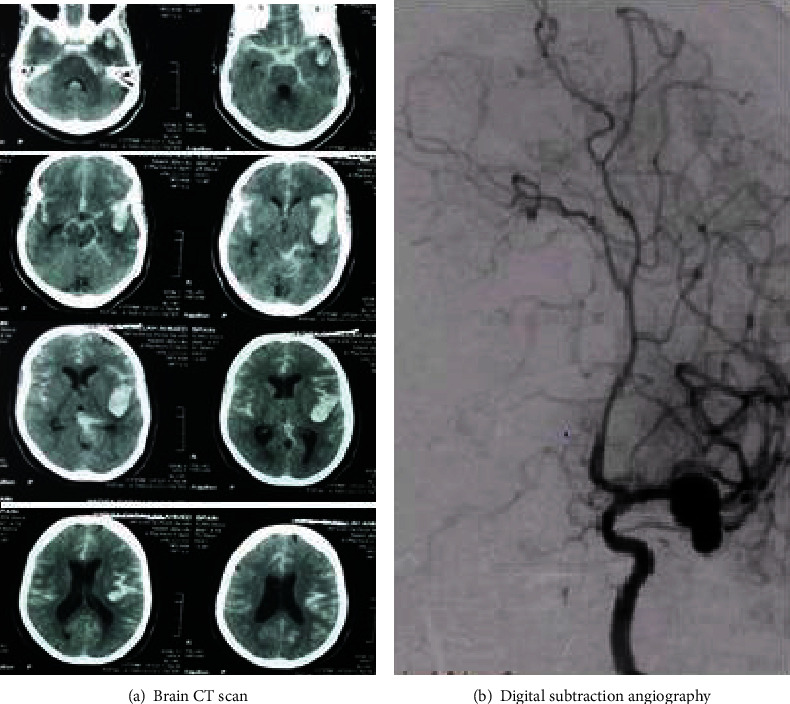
Diagnosis of aSAH by brain CT scan and digital subtraction angiography. (a) Brain CT scan was done revealing subarachnoid hemorrhage. Hunt and Hess scale was III. (b) Digital subtraction angiography was done showing the left MCA aneurysm.
